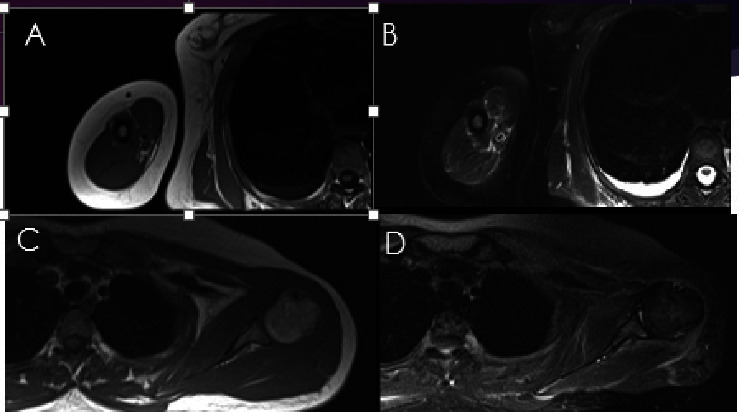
MRI of right arm T1W (a) and fat sat (b) and (c)-(d) MRI of left shoulder T1W (c) and fat sat (d) sequences. Note to the diffuse perimuscular and fascial edema more along the latissimus dorsi and rotator cuff muscles (arrows).
